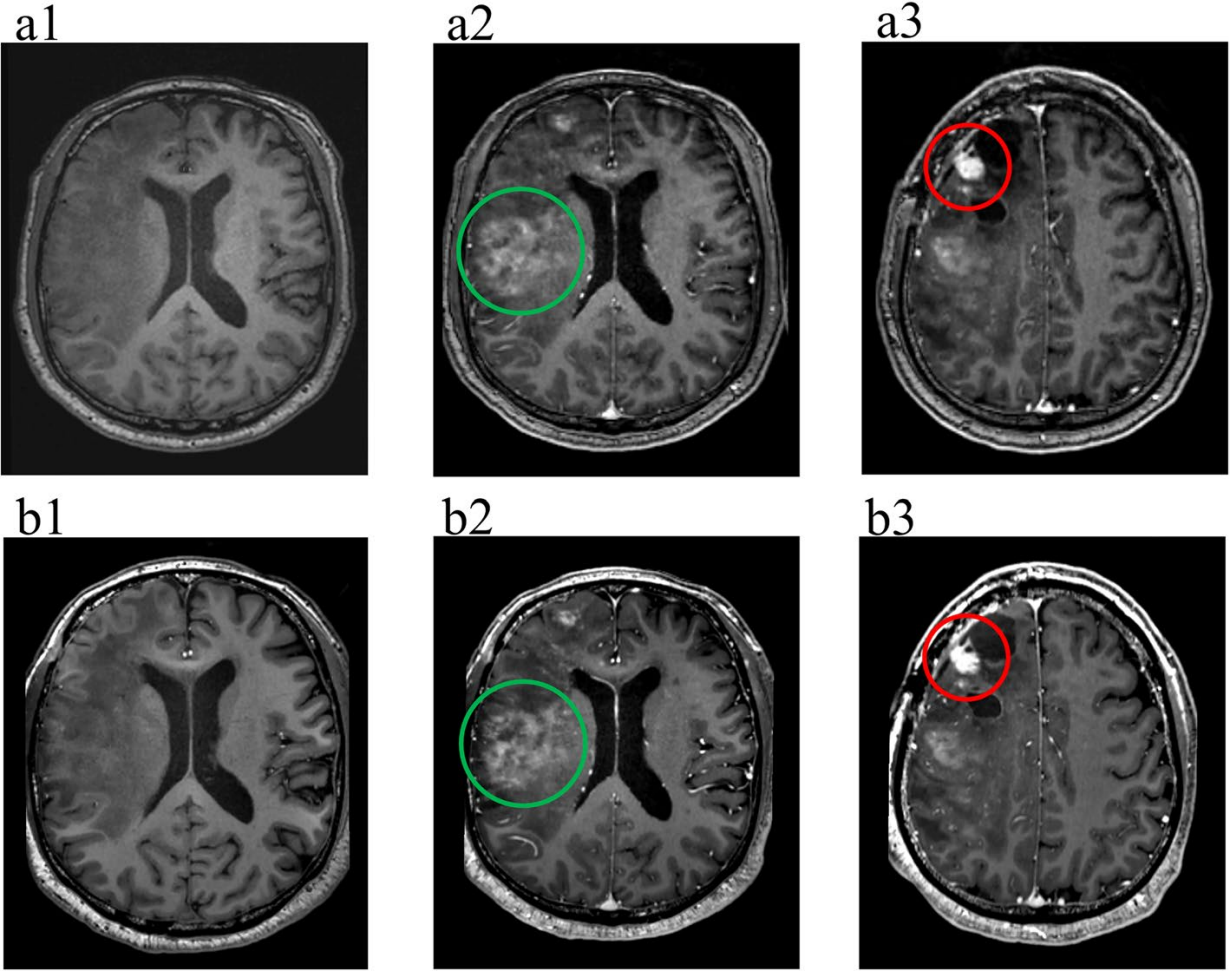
Anaplastic oligodendroglioma, WHO Grade III, 49-year-old male, relapsed after surgery in 2014. (a1) T1w MRI without contrast enhancement at 3.0 T and (b1) T1w MRI without contrast enhancement at 5.0 T. (a2) and (a3) Two different slices of full-dose enhanced T1w MRI at 3.0 T. (b2) and (b3) Two different slices of half-dose enhanced T1w MRI at 5.0 T. For lesions with low-level blood‒brain barrier (BBB) leakage (green circle), the enhancement effect was similar between 3.0 T and 5.0 T, but for lesions with high-level BBB leakage (red circle), the enhancement effect with a half-dose at 5.0 T was significantly better than that with a full-dose at 3.0 T
